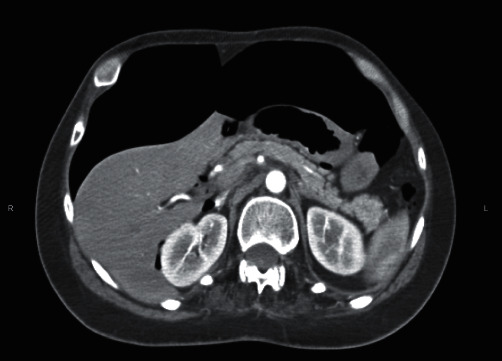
Computed tomography (CT) scan of the chest, abdomen, and pelvis (axial view).
